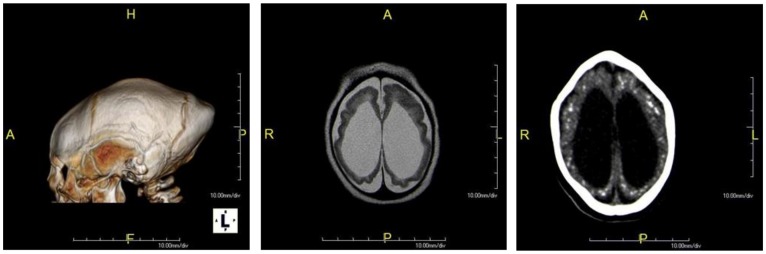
Neuroimage findings in infants with Congenital Zika Syndrome (CZS). 3D CT image of a 3-month-old infant with probable CZS evidencing microcephaly and prominent occiput (A). Axial T2/FSE (Fast Spin Echo) MR image of a 4-month-old infant with possible CZS demonstrating ventriculomegaly, diffuse cerebral atrophy, and malformations of cortical development with a simplified gyral pattern (B). Axial CT image without contrast of a 4-month-old infant with possible CZS evidencing microcephaly, ventriculomegaly, and bilateral cortical and subcortical calcifications (C).
